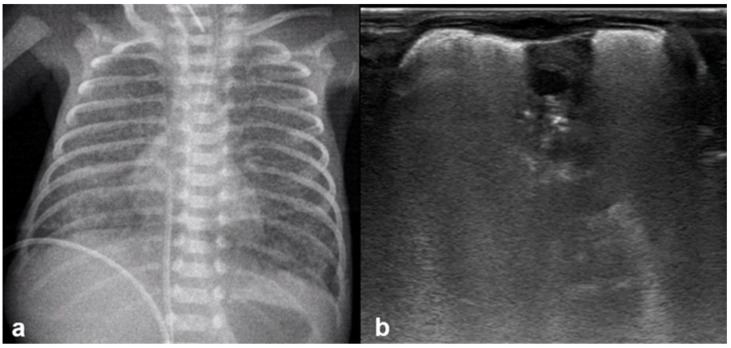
Interstitial emphysema shown on chest x-ray (a) and LUS (b). This case underlines the superiority of CXR in differentiating this pathology from RDS, which has a similar LUS appearance.
